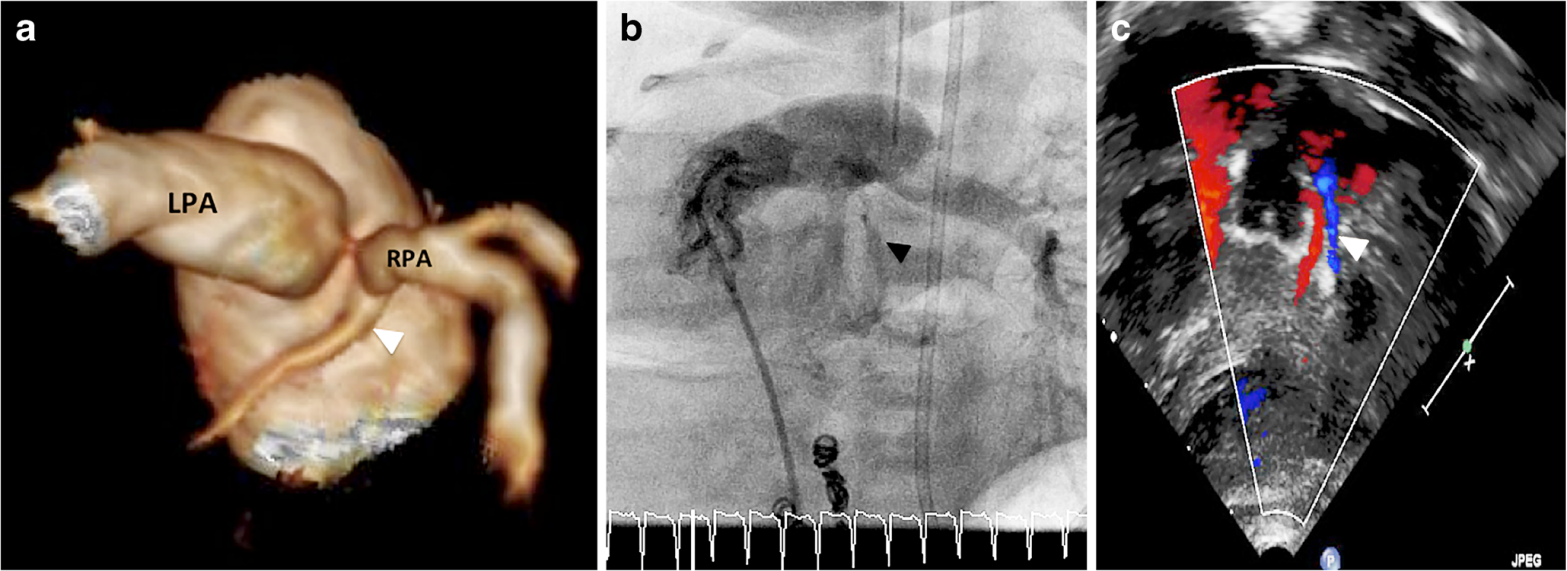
Main imaging findings. a-c A female neonate with antenatally diagnosed scimitar syndrome undergoing CT for investigation of unexpected dyspnoea. Posterior projection volume-rendered tomogram (a) from an ungated high-pitch CT demonstrates anomalous origin of the left circumflex coronary artery (*arrowhead*) immediately inferior to the origin of the hypoplastic right pulmonary artery (RPA) The left pulmonary artery (LPA) is normal. Invasive catheter angiography (b) confirms the anomaly (*arrowhead*). Note the embolisation coils in the systemic arterial collaterals. The anomaly was subsequently also demonstrated via echocardiography (c). Retrograde flow was demonstrated in the left circumflex coronary artery (flow in blue marked by an *arrowhead)*. Antegrade flow is demonstrated in red in the adjacent left anterior descending artery
